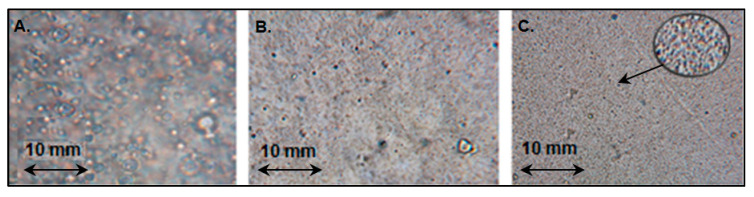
Photographs of the pPVC films produced without sonication and the film loading at 20 °C (A) and using ultrasonic irradiation followed by film loading at 20 °C (B) and 60 °C (C).
